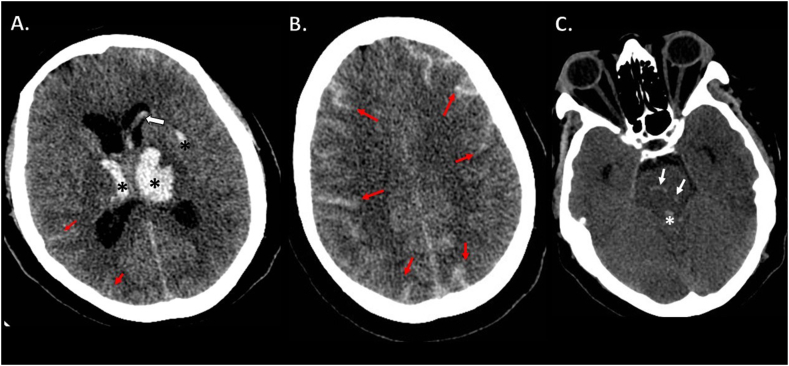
CT scans of bilateral thalamic hemorrhages. A: axial CT scan at thalamic level depicts bilateral thalamic and left external capsule hemorrhages (black stars), intraventricular propagation (white arrow) and subarachnoid hemorrhage (red arrows). B: axial CT scan at centrum semiovale level shows extensive subarachnoid hemorrhage components (red arrows). C: axial CT scan depicts small hemorrhages in the mid-pons region (white arrows) and hemorrhage in the 4th ventricle (white star).
